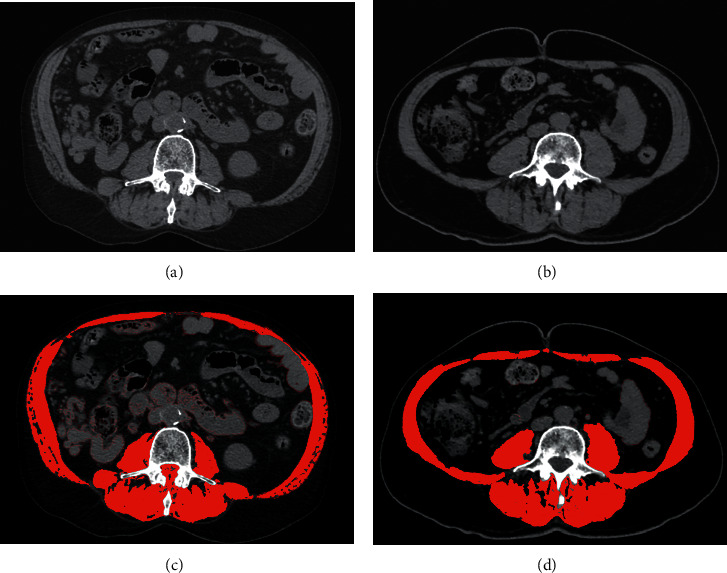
Computed tomography images of two different patients, one affected by HCC (a) and one affected by pancreatic cancer (b). Both patients demonstrate imaging sign of sarcopenia using the public domain software (ImageJ) (B B').
